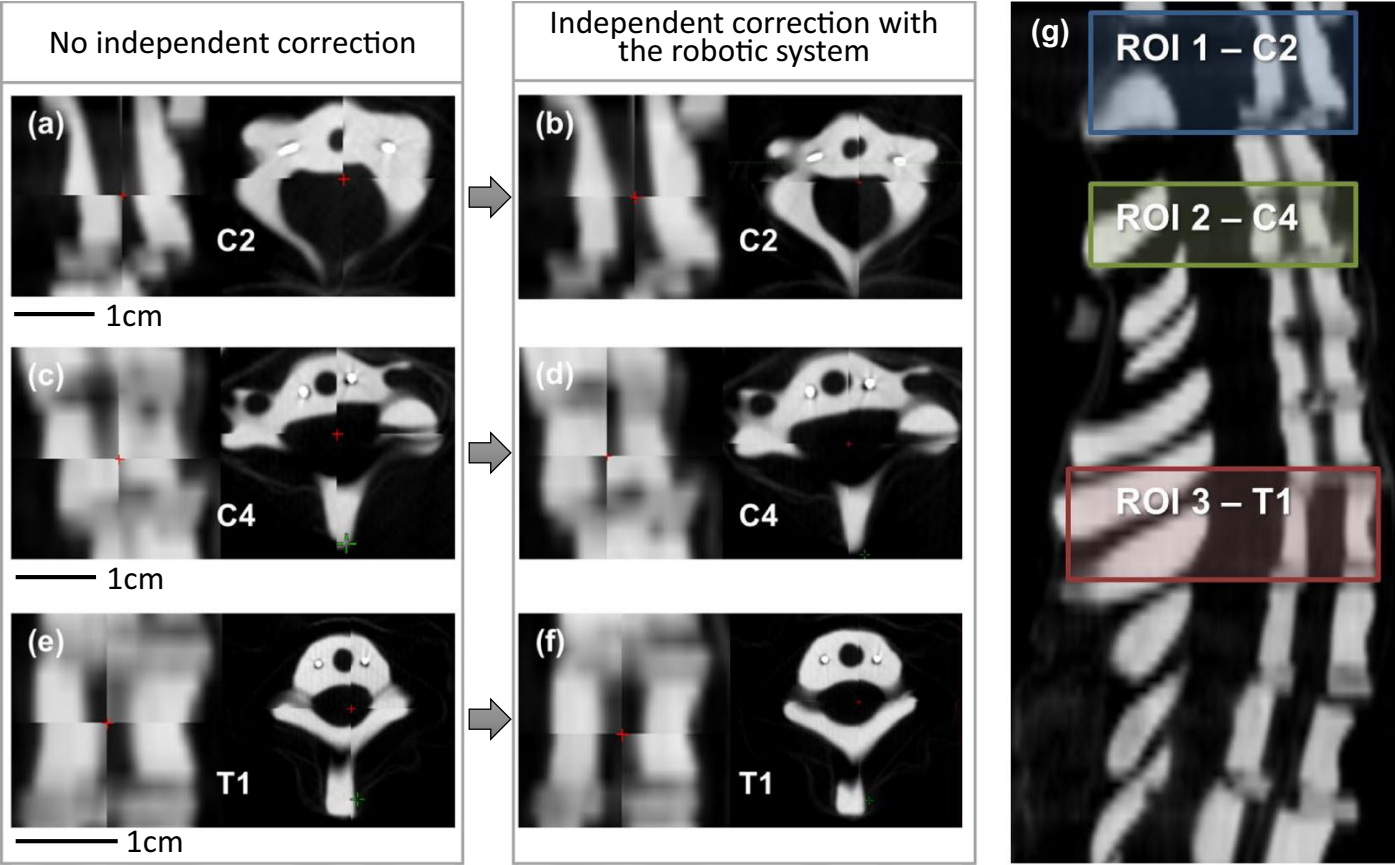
Comparison of no independent correction of CBCT images with the case of the robotic system-assisted correction. (a,c,e) CBCT images without independent correction for C2, C4, and T1, respectively. (b,d,f) CBCT images that are independently corrected by the robotic system for C2, C4, and T1, respectively. (g) Regions of interest of C2, C4, and T1 in a skeleton figure.
